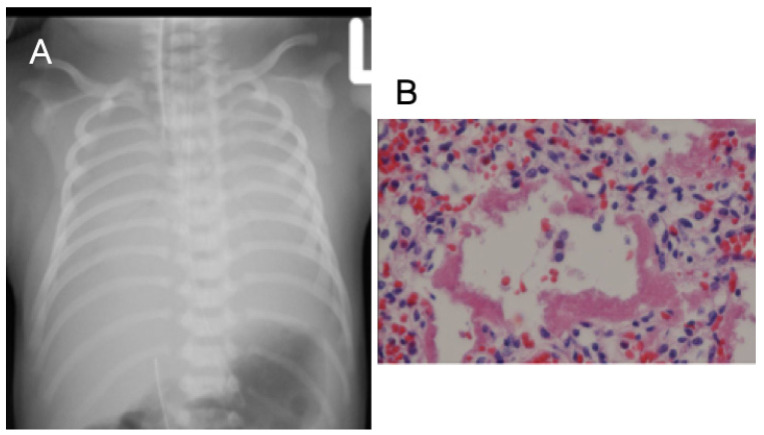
(A) Chest radiography of a male neonate presenting with cyanosis and pulmonary hypertension, revealing diffuse ground glass lungs with low volumes; (B) lung section showing that the alveolar ducts, alveoli, and terminal and respiratory bronchioles are lined with an eosinophilic hyaline membrane with necrotic alveolar lining cells and fibrin (40×).
